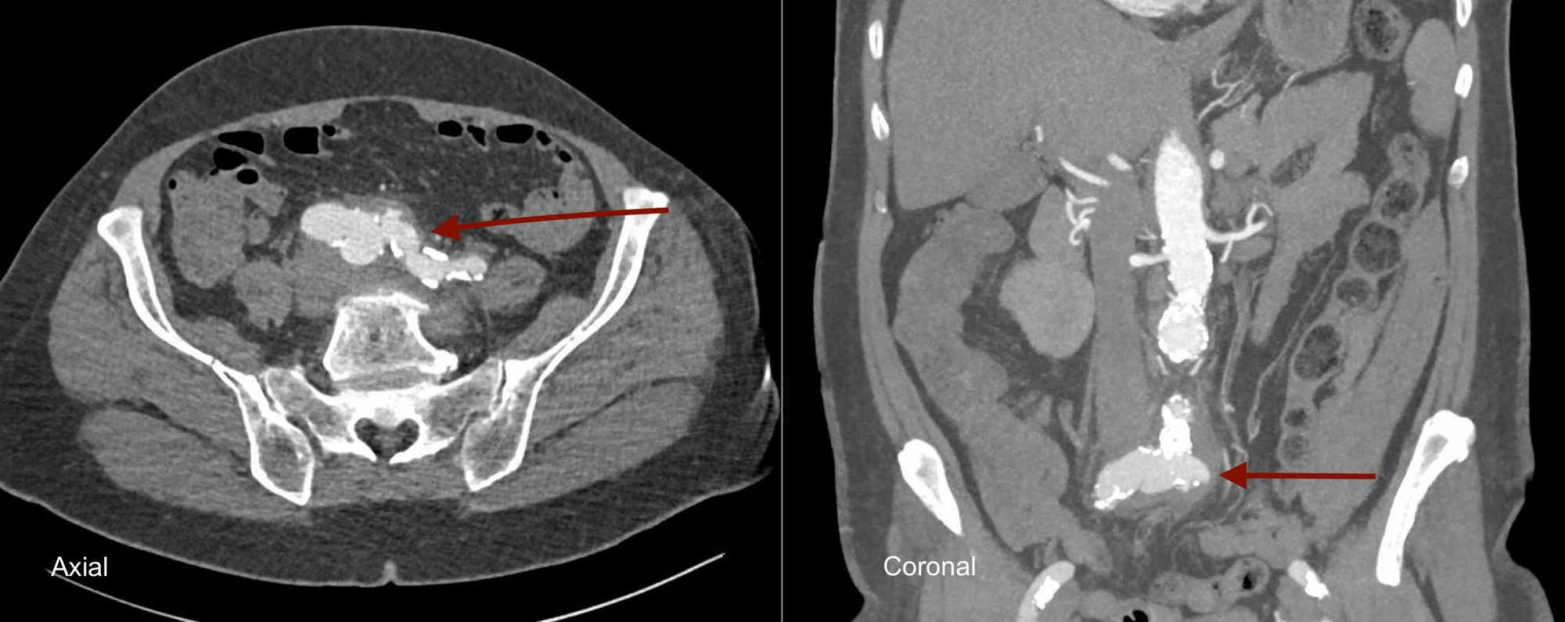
Mycotic aneurysm of aortic bifurcation (axial and coronal contrast-enhanced computed tomography)
